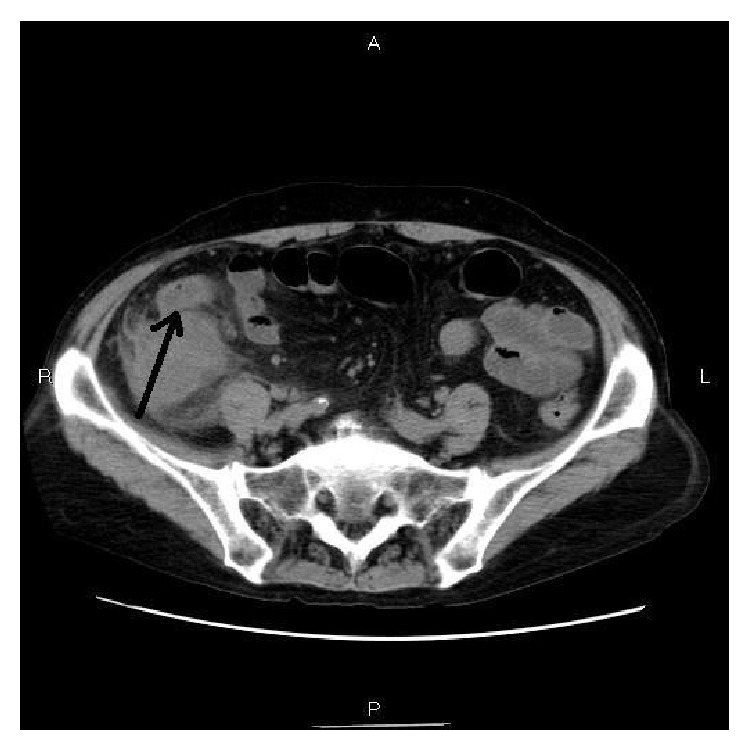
Abdominal CT. The appendix shows a mass measuring 5 × 4 × 4 cm. The appendix cannot be identified (arrow).
